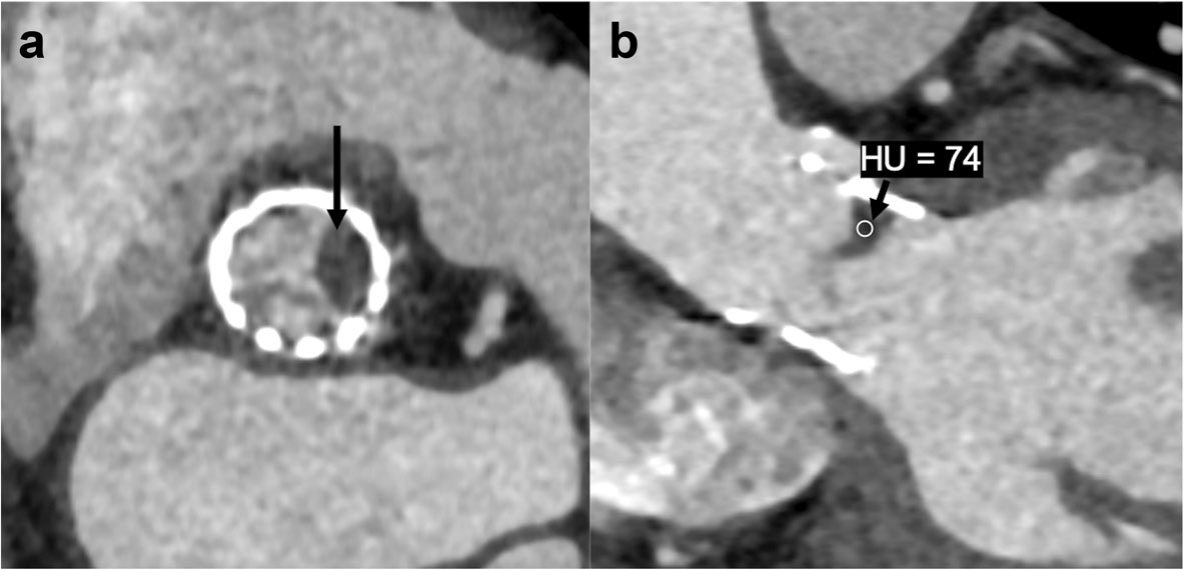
A 67-year-old female status transcatheter aortic valve replacement (TAVR) using Sapiens valve (Edwards Lifesciences) with suspected aortic valve lesion on echocardiogram. Axial (a) and coronal oblique (b) reconstructed CTA images reveal biconvex hypoattenuating (Hounsfield unit, 74) leaflet and left cusp thickening causing restricted motion (seen on cine), consistent with thrombus
